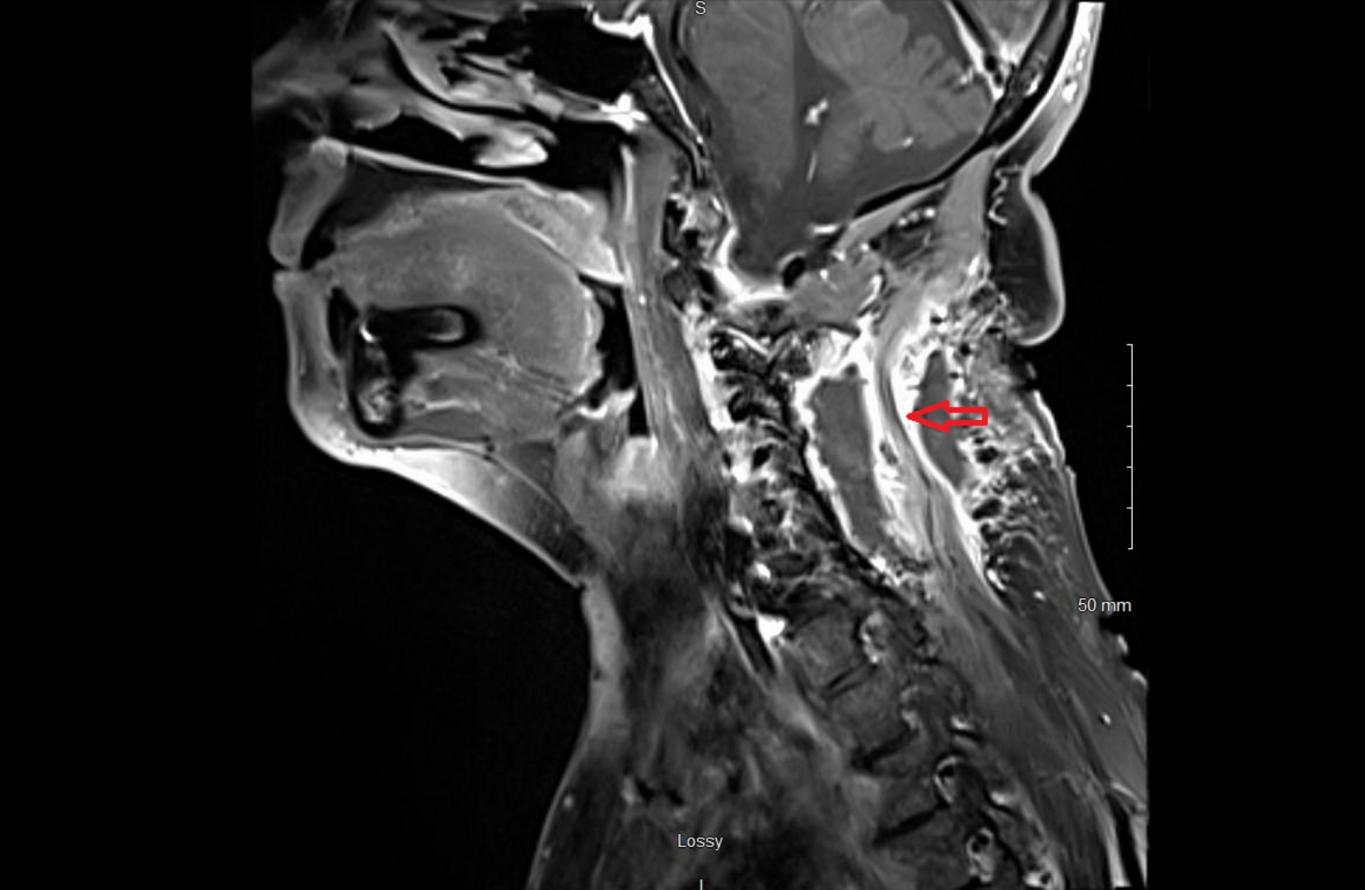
Parasagittal MRI postsurgical resection. T1 weighted enhanced MRI of the cervical spine four weeks after resection, showing gross total resection with associated postoperative changes in the parasagittal plane.
